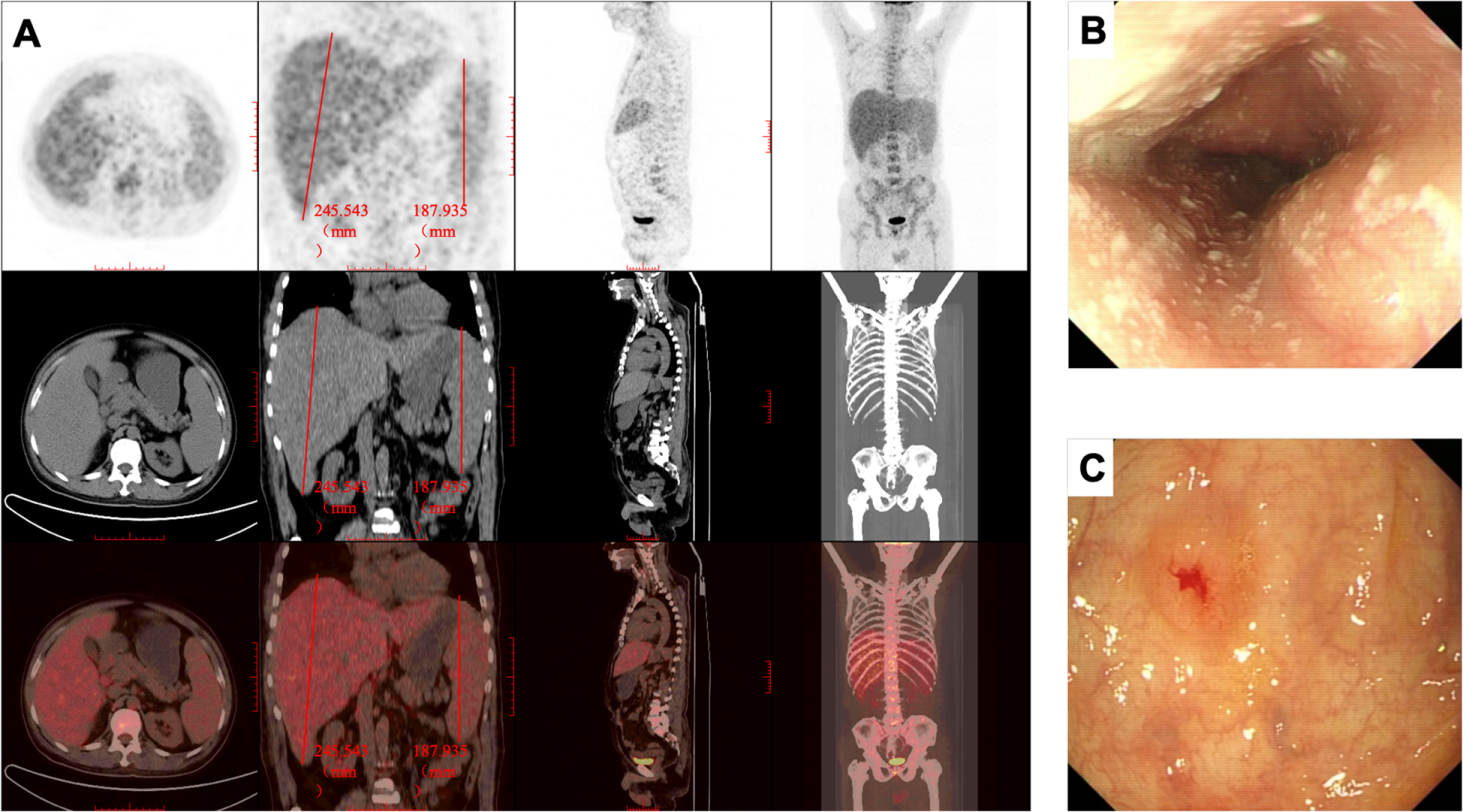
a Increased FDG uptake in liver and spleen; the length of liver was 245.543 mm and the length of spleen was 187.935 mm in the ^18^F-FDG PET/CT images before intervention. b esophageal mucosa was covered consecutive sheets of white bean curd-like substance under gastrointestinal endoscopy. c colon mucosa studded with protuberant lesions with central depression and erosion under gastrointestinal endoscopy
